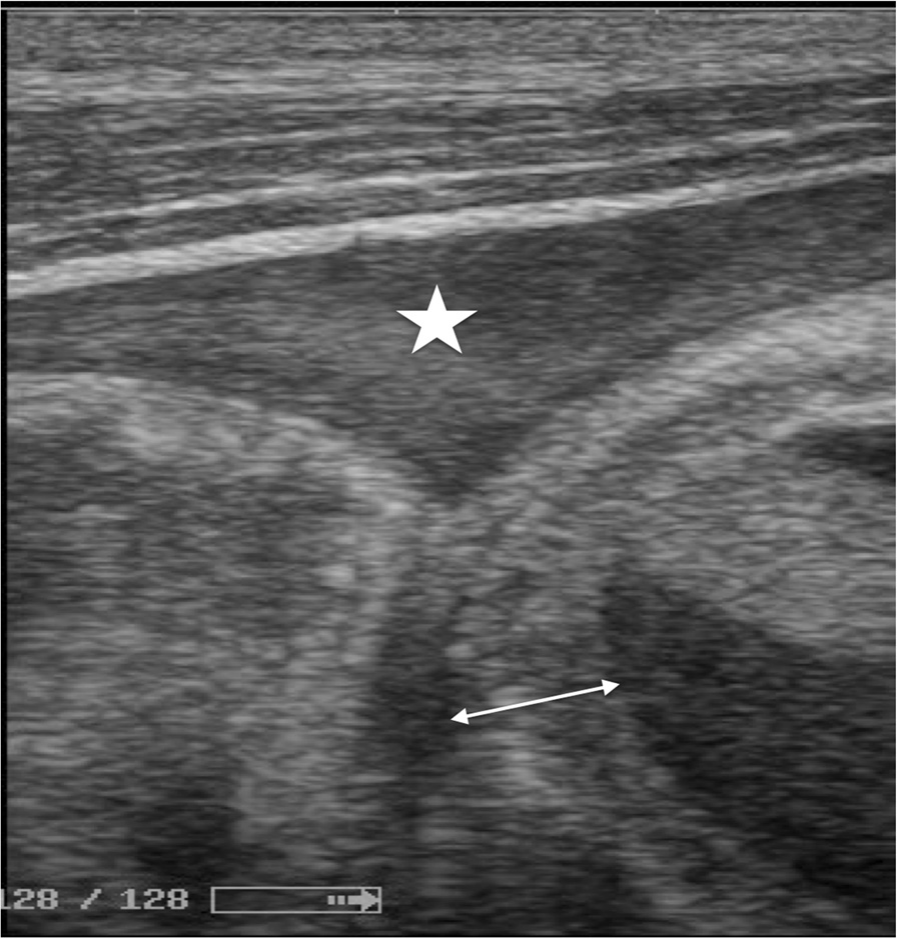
Ultrasound image of a hemoperitoneum (star) in the right paracolic gutter with thickened colonic walls suggesting a parietal hematoma (double-headed arrow)
